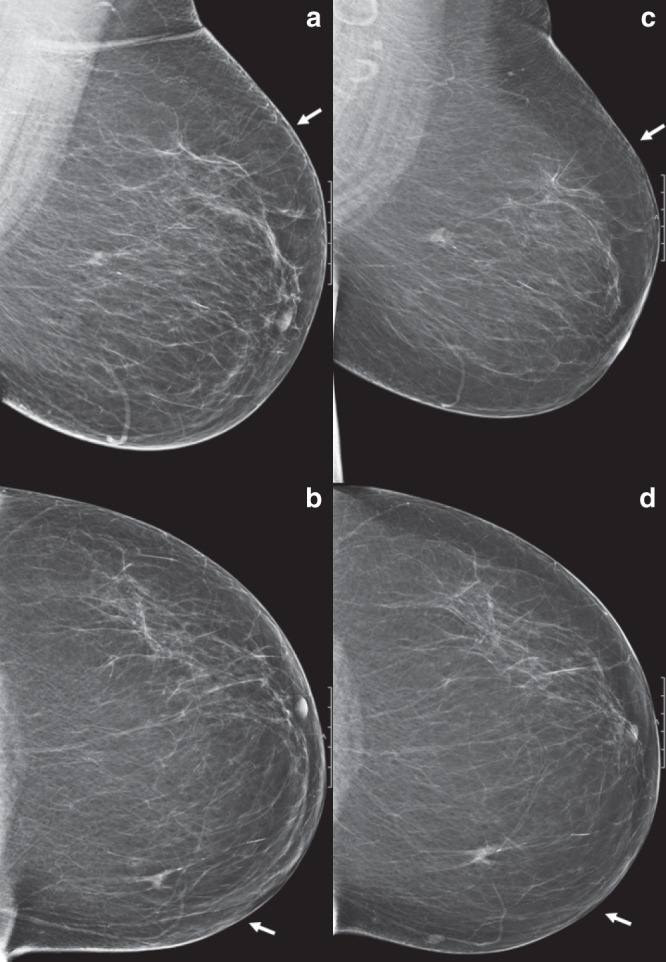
Patient example, repeated recall after previous false-negative recall. Two-view screening mammograms (a and c, medio-lateral oblique (MLO) view, and b and d, cranio-caudal (CC) view) of the left breast in 2014 (a and b) and in 2016 (c and d). In 2014, it shows a lesion in the medial upper quadrant of the breast (arrows), initially classified as BI-RADS 0 (additional analysis needed) by the screening radiologist. At recall (2014), additional digital breast tomosynthesis (DBT) and ultrasound were performed, and the lesion was classified as BI-RADS 2 (benign lesion). No biopsy was performed. Two years later (2016), the patient was recalled for the same lesion now classified as BI-RADS 5 (due to spiculae). Ultrasound-guided true-cut biopsy was performed, which revealed an invasive lobular carcinoma without axillary metastases. The patient was treated with breast conserving surgery and adjuvant radiotherapy.
